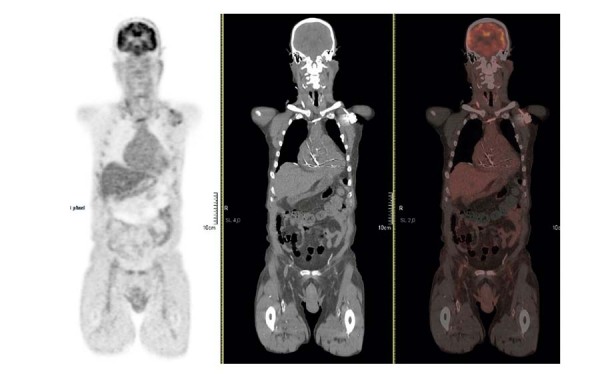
Imagens coronais de corpo inteiro obtidas por PET/CT com 18F-FDG (PET, CT e fusão, da esquerda para a direita) mostram captação focal ao longo do eletrodo e gerador do marca-passo.
